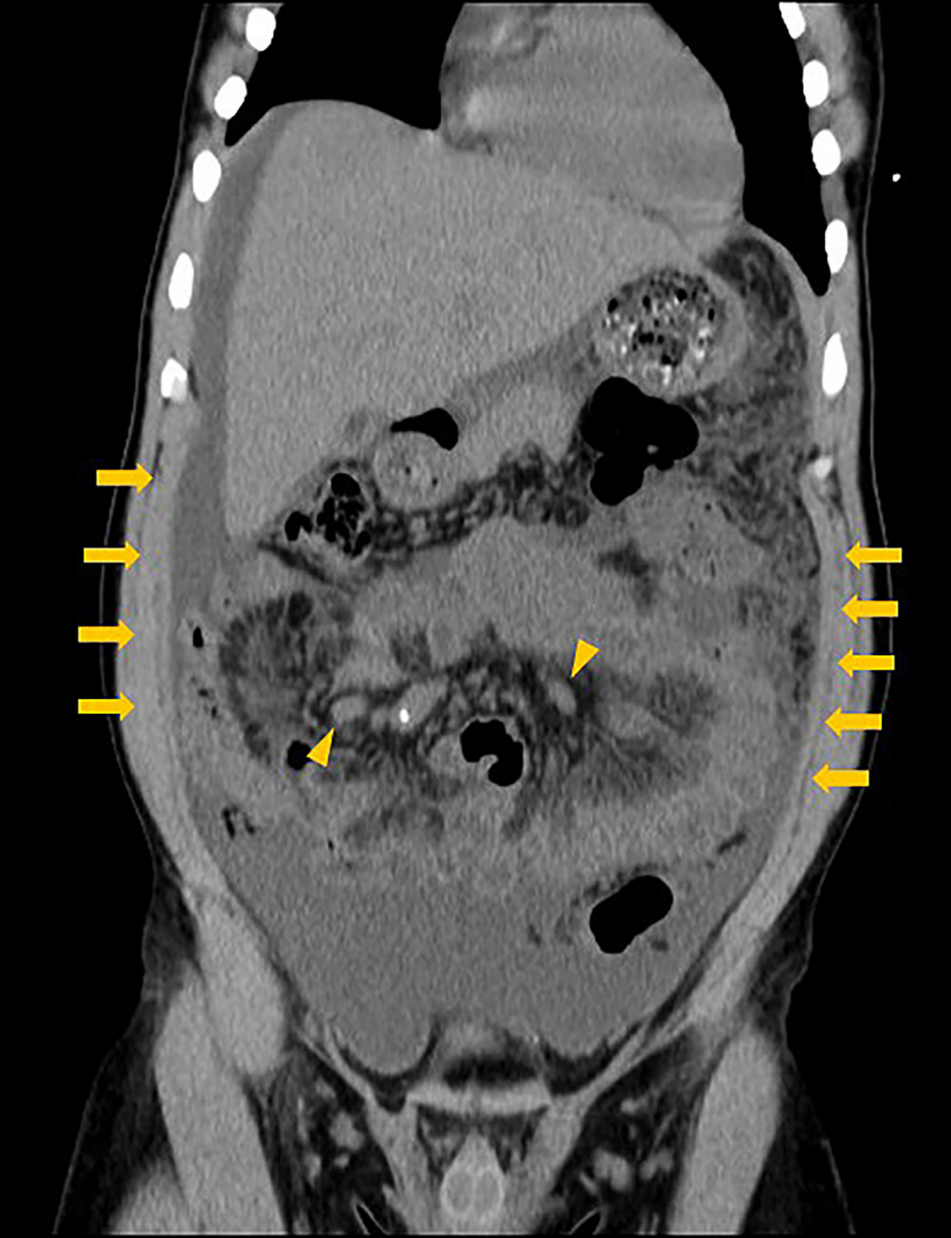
Findings of abdominal contrast‐enhanced computed tomography on admission. There is massive ascites, diffuse, smooth, homogeneous thickening of the peritoneum (arrows) and enlarged mesenteric lymph nodes (arrowheads).
